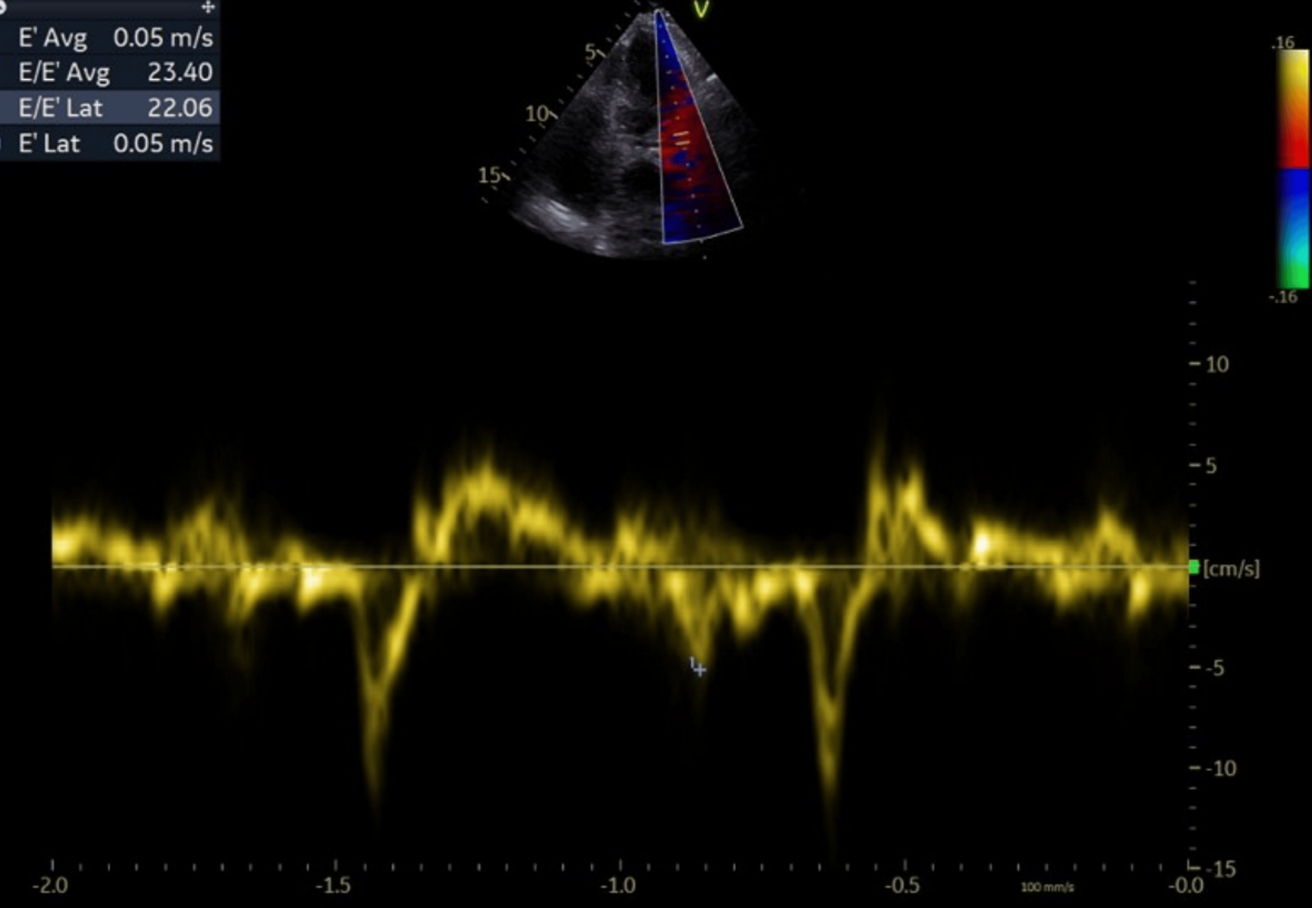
Evidence of elevated end-diastolic pressure of the left ventricle (E/E’ avg=23.4). A further increase of this ratio indicates a deterioration of the diastolic dysfunction. The E wave is the early diastolic filling wave seen on Doppler interrogation of the mitral valve. The E' wave is acquired in tissue Doppler either of the basal or the lateral wall (in our case we used the mean value) of the mitral annulus. Values greater than 15 suggest that left ventricle end diastolic pressure is elevated.
